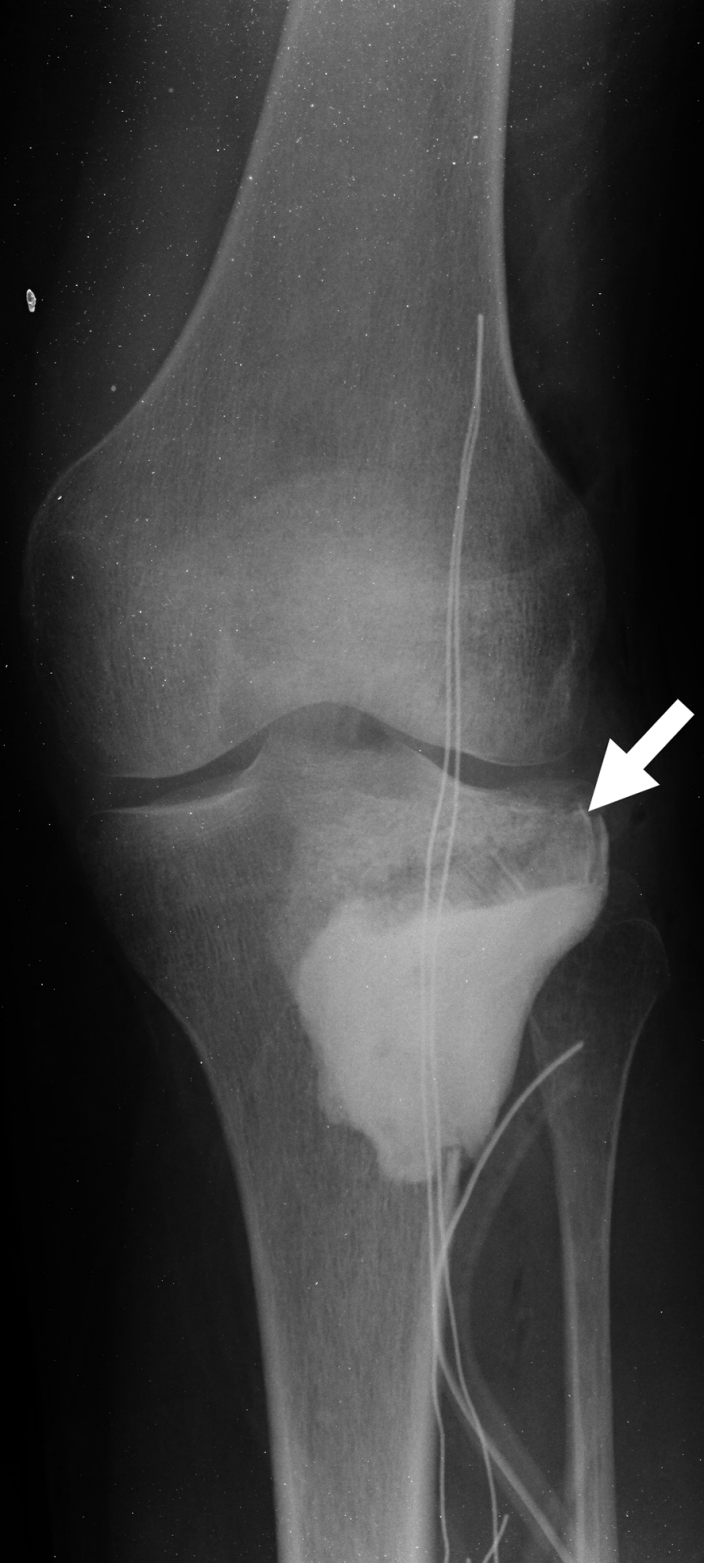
Radiography after curettage followed by filling with bone cement after allograft chip bone filling in the subchondral region. The arrow indicates the allograft chip bone.
